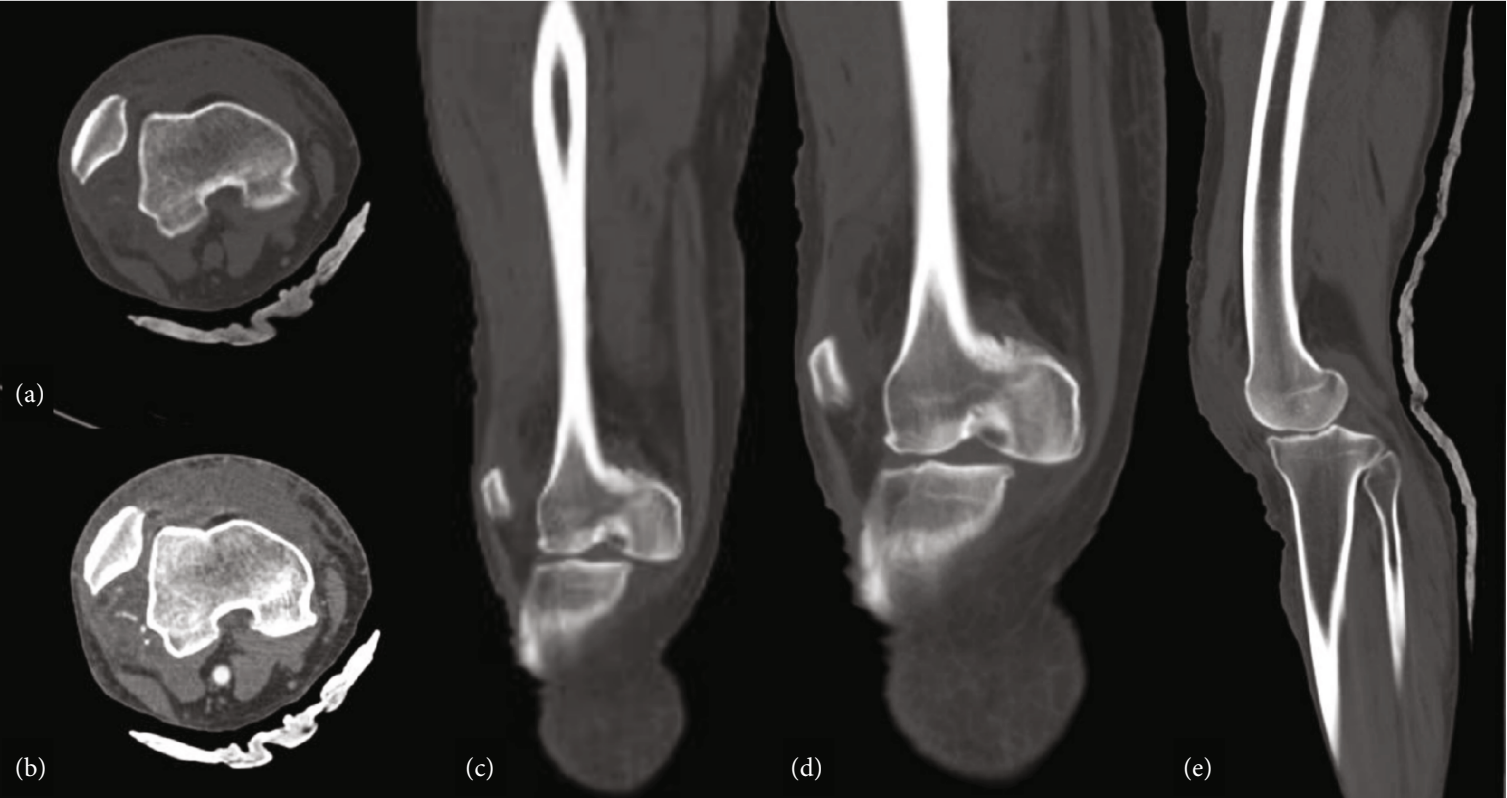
(a, b) Axial, (c, d) AP, and (e) lateral CT-scan: dislocation of the femorotibial joint is observed, with medial displacement of the femoral portion. Additionally, an avulsion fracture is identified on the lateral tibial surface, specifically in its medial portion. Furthermore, lateral displacement of the patella is noted. The quadriceps and patellar tendons exhibit normal density.
